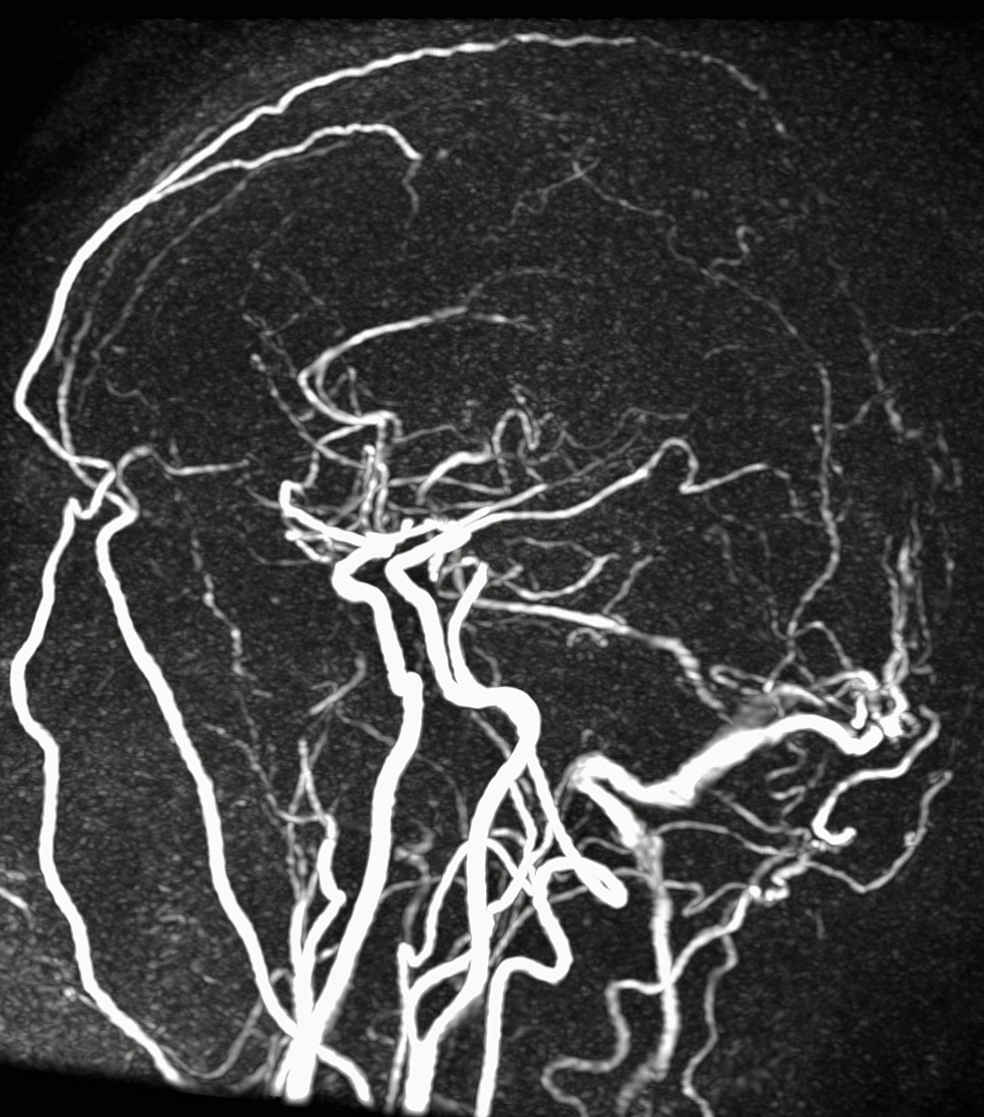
MRI Brain Venogram - Cerebral Venous Sinus Thrombosis A non-invasive diagnostic procedure that uses a combination of a large magnet, radio frequencies, and a computer to produce detailed images of organs and structures within the body without the use of damaging ionizing radiation. The mid and posterior parts of the superior sagittal sinus show no flow in venography. There is non-visualization of flow in the right transverse, sigmoid sinus, and jugular bulb. The proximal aspect of the left transverse sinus also shows partial loss of flow. No obvious flow was seen in Galen's straight sinus and vein- these findings suggest cerebral venous sinus thrombosis.
